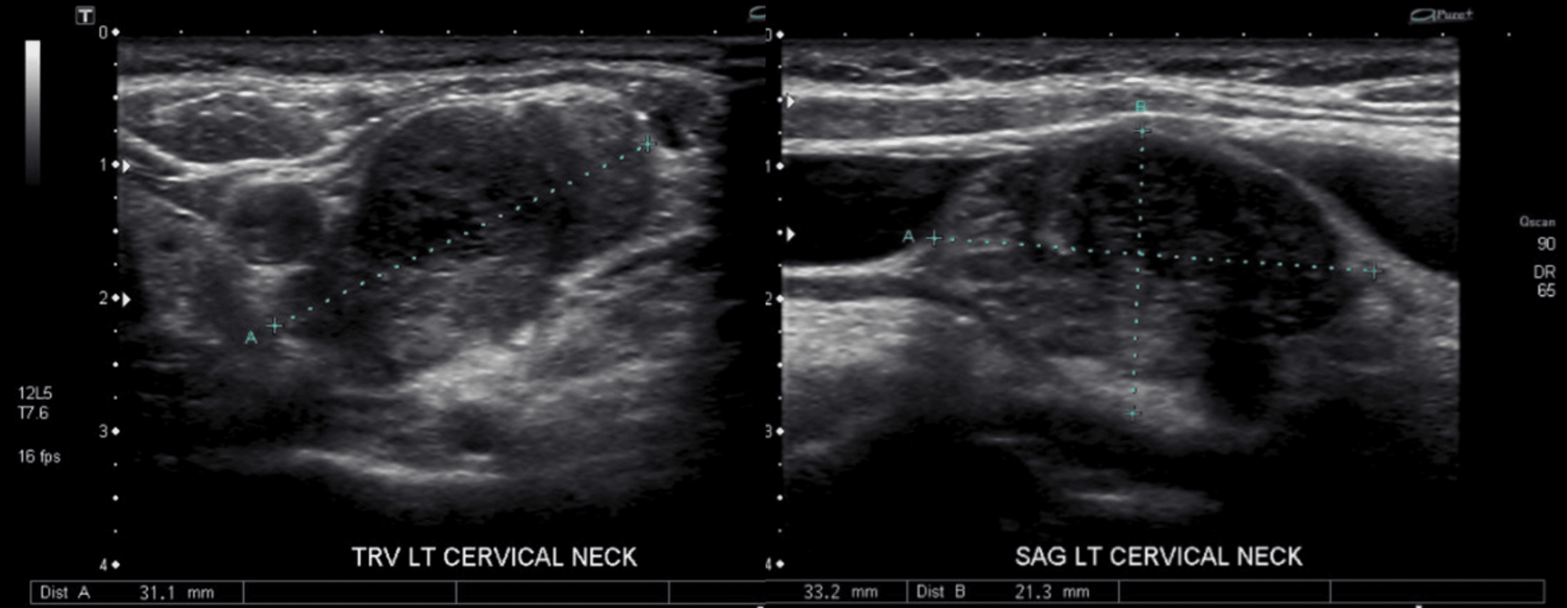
Ultrasound imaging of left cervical neck mass: transverse (left) and sagittal (right) views
